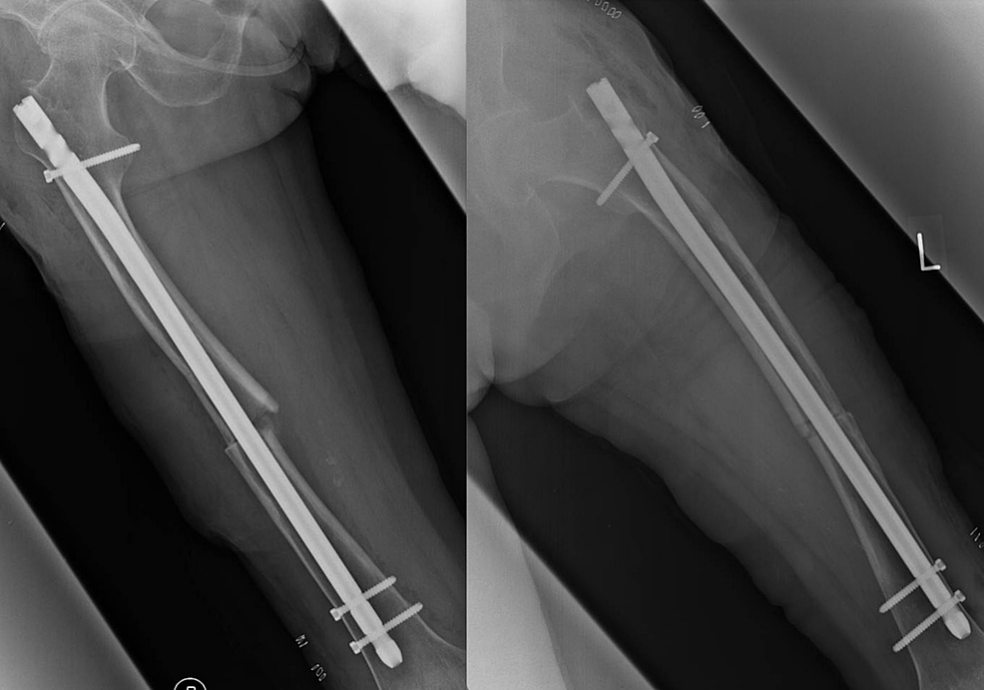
Plain radiographs of the right and left femurs immediately after surgery.
